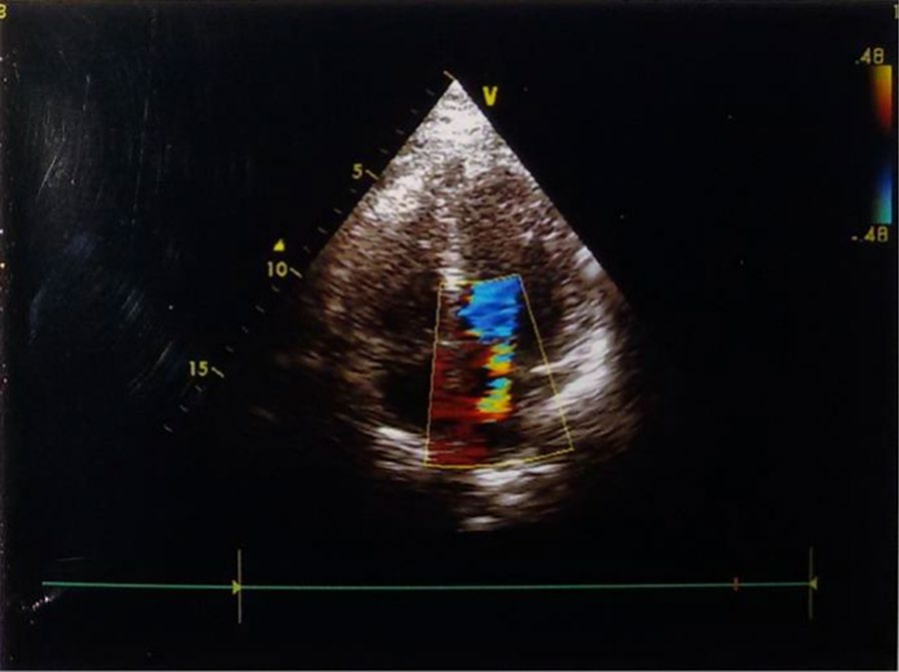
Color Doppler echocardiography parasternal long axis view showing moderate mitral regurgitation
